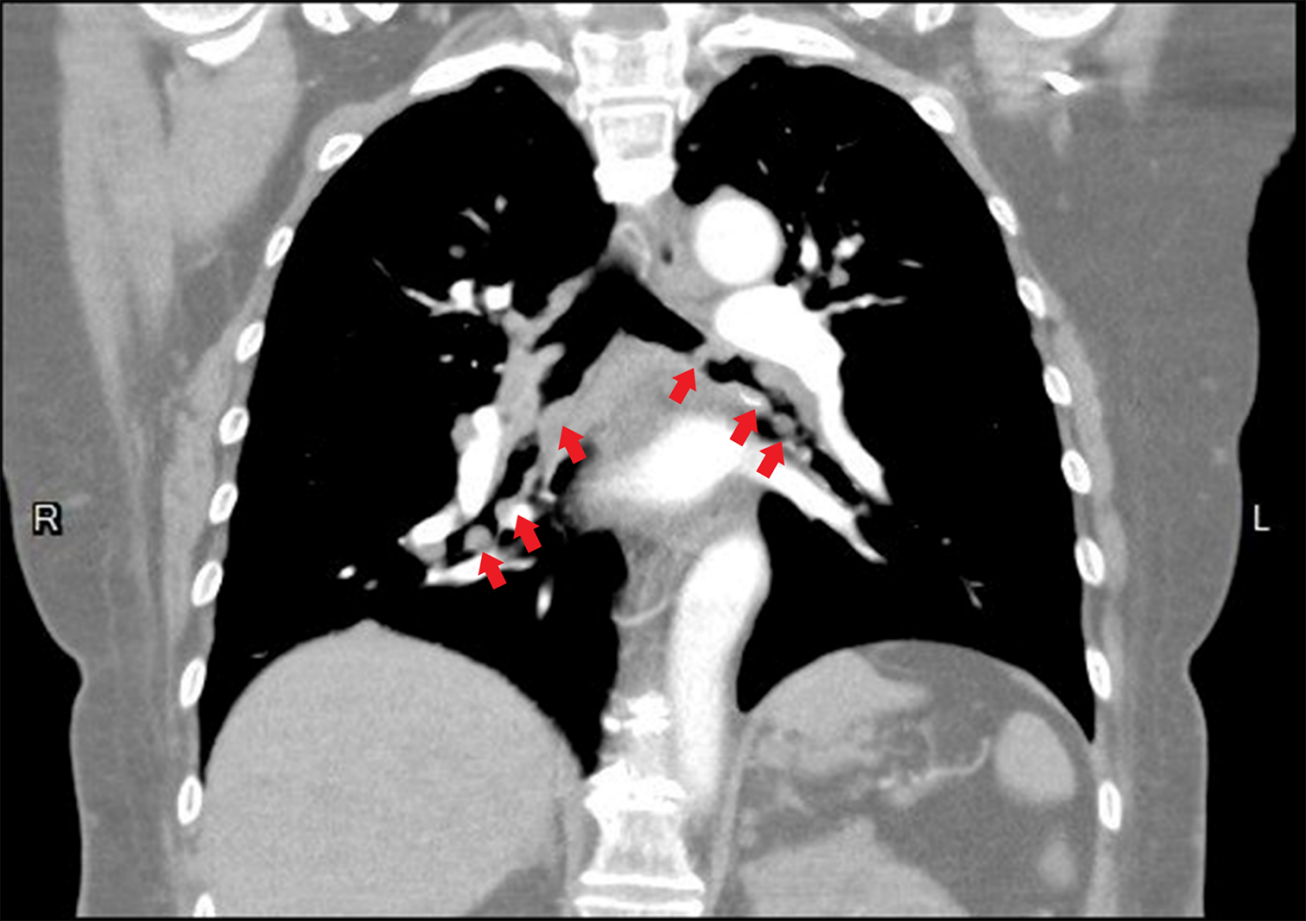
Contrast chest CT showed multiple tracheobronchial nodules in primary and secondary bronchus (Coronal view).
